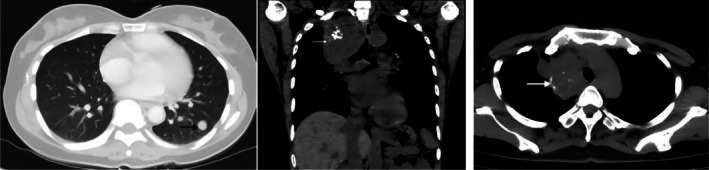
(4) CT image shows a metastatic nodule in the left lower lobe (→). (5) Follow‐up CT after anlotinib therapy shows reduction in the right upper lobe mass (→) and metastatic nodules (→).
